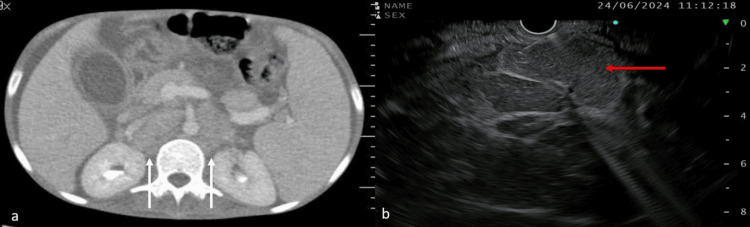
a) Contrast-enhanced computed tomography image showed multiple aorto-caval and paraaortic lymph nodes (white arrow); note is made for ascites. (b) Endoscopic ultrasound image shows an enlarged aorto-caval lymph node (red arrow).
